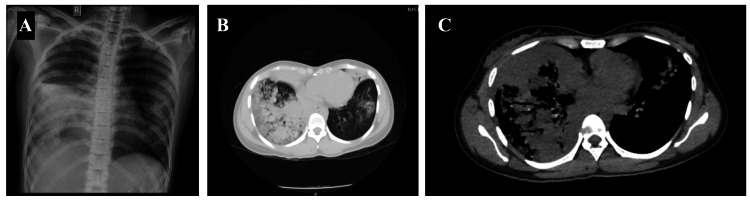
Radiological findings on admission. (A) Initial chest radiograph obtained on admission (day eight of illness) showing homogeneous opacity in the right mid and lower zones silhouetting the right cardiac border (silhouette sign), right costophrenic recess, and right hemidiaphragm without any tracheal or mediastinal shift. Multiple non-homogeneous opacities noted in the left mid and lower lung zones. (B) High-resolution computed tomography of the chest showing large areas of lobar consolidation involving the right middle lobe, superior and basal segments of the right lower lobe with air bronchogram, and a few patchy areas of consolidation in both lungs. (C) High-resolution computed tomography of the chest (mediastinal window) depicting mediastinal lymphadenopathy.
